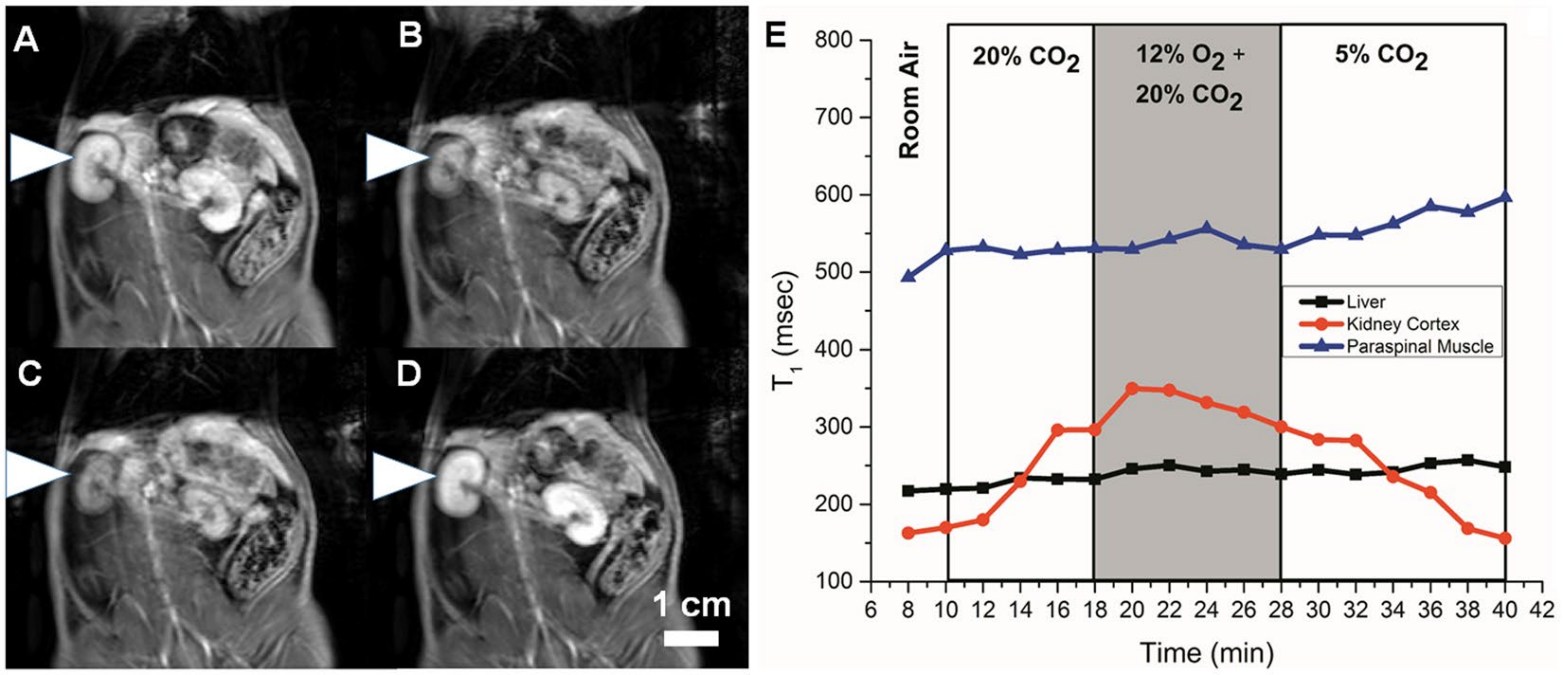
Dynamic MR imaging of hypercapnia- and hypercapnic hypoxia-mediated vasomotion. The kidney is shown on *T*
